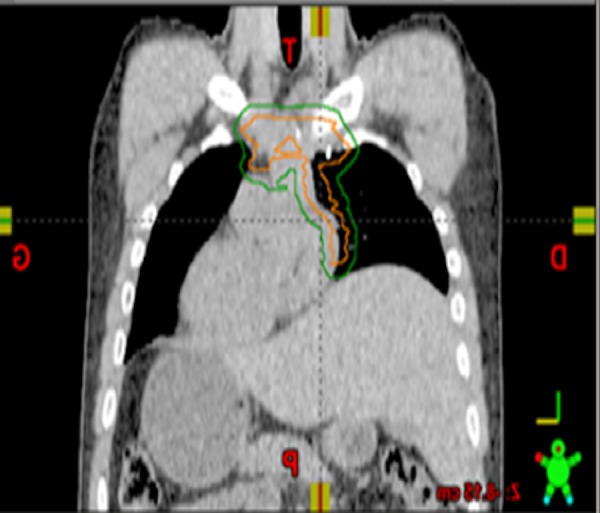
Coronal computed tomography image from the radiotherapy planning study with an outline of the clinical target volume (ORANGE) and the planning target volume (GREEN).
